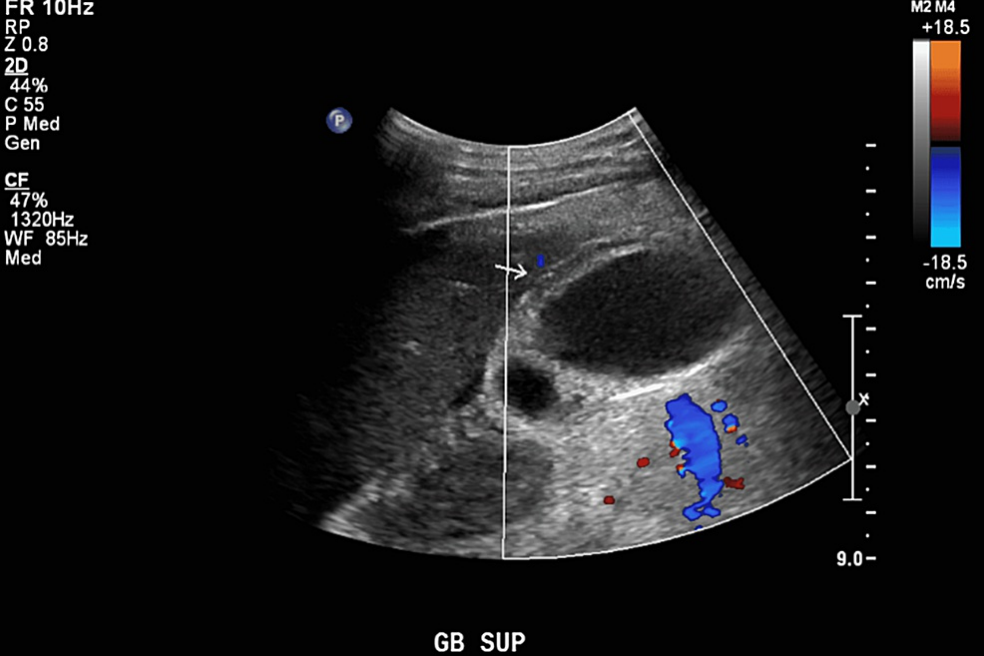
Ultrasound (US) of right upper quadrant depicting a distended gallbladder (arrow).
